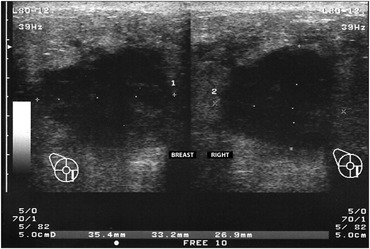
Breast ultrasound showing an irregular lesion with a central cavity containing liquid material.
